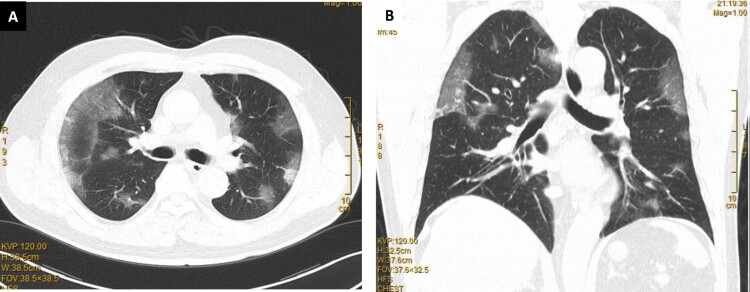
Typical changes of COVID-19 pneumonia on lung computed tomography showing bilateral multifocal patchy ground glass opacities: (A) transverse view; (B) coronal view.
